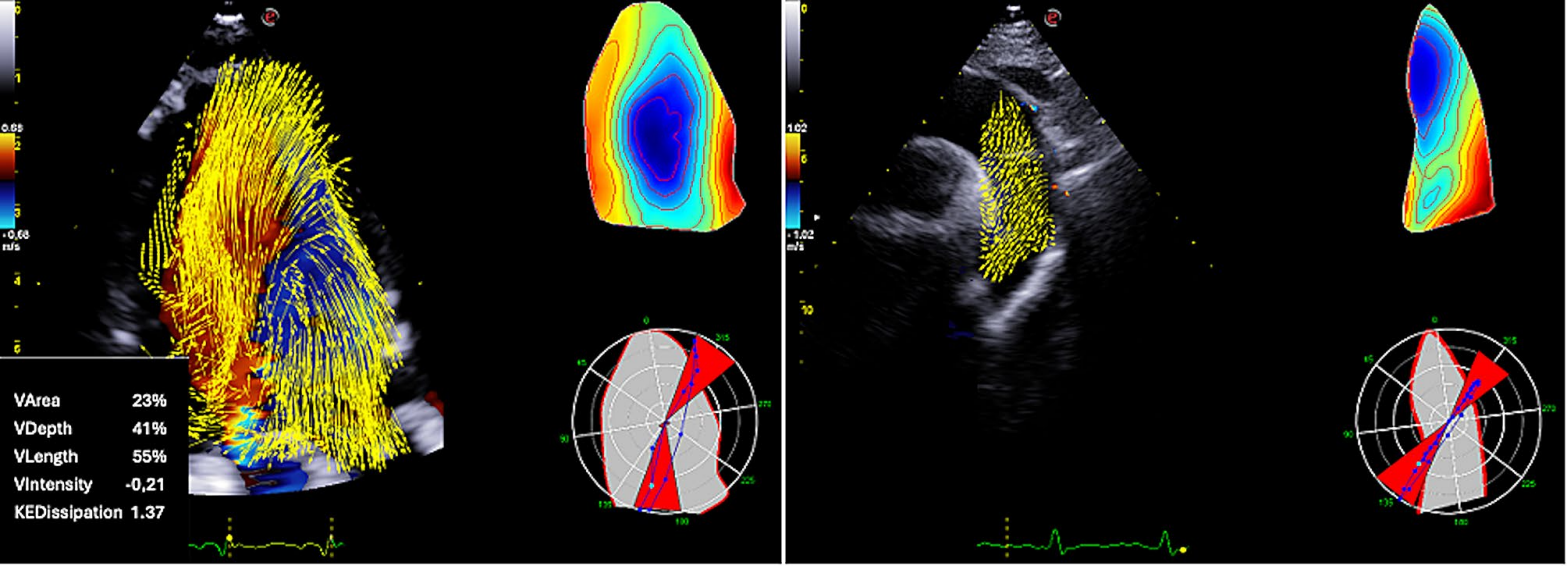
Intracardiac flow analysis using vector flow mapping. Fluid dynamic evaluation using the HyperDoppler Software in a patient with interventricular septal defect (on the left). The software allows both qualitative (polar and vector maps) and quantitative assessment of main vortex parameters. On the right, evaluation of the aortic arch using the same technique
